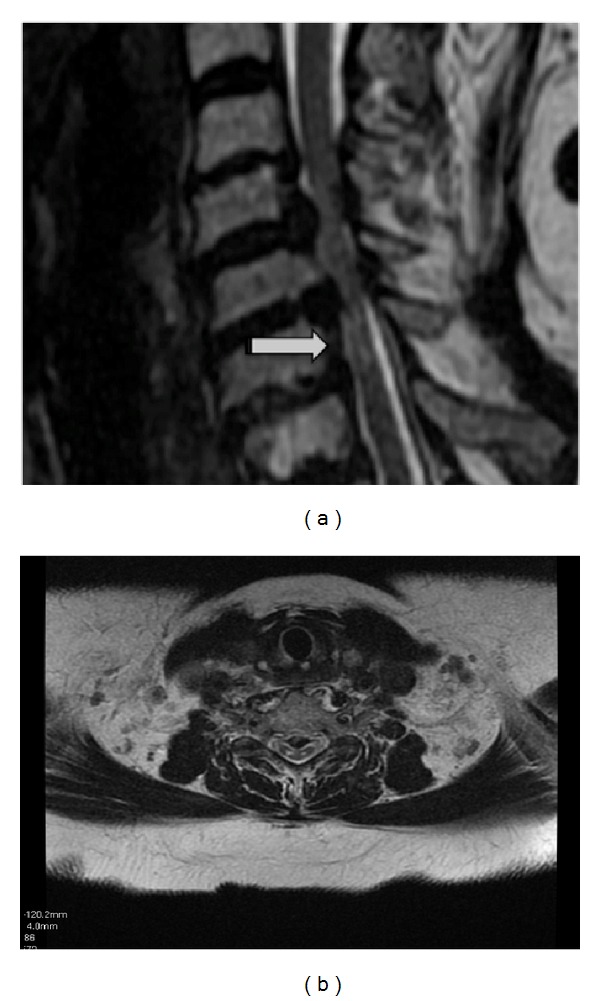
(a) and (b): T2-weighed MRI on sagittal and axial plans of spinal cord linear nonenhancing high signal intensity lesions.
